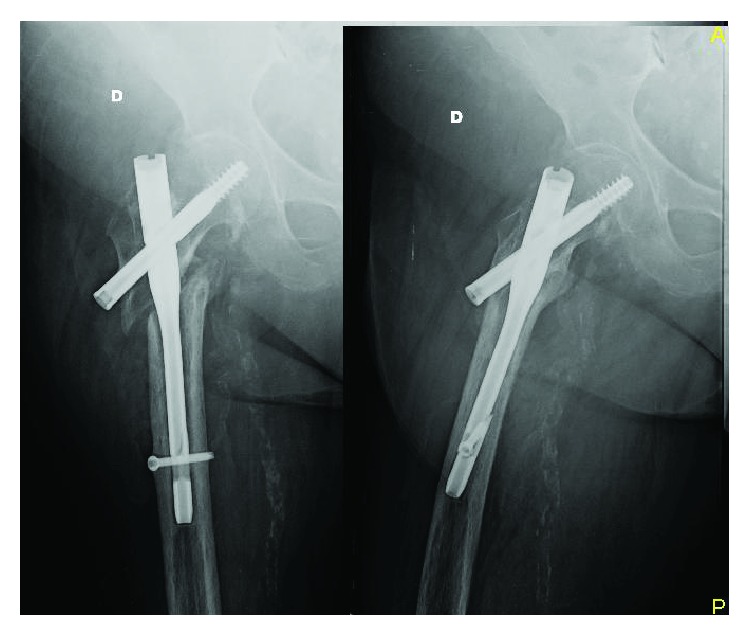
X-rays done in other hospital after 1 year of follow-up showed that the first incomplete breakage was on the hole for the distal static screw. The orthopaedic surgeon did not talk about nail dynamization or nail remotion and revision to the patient.
